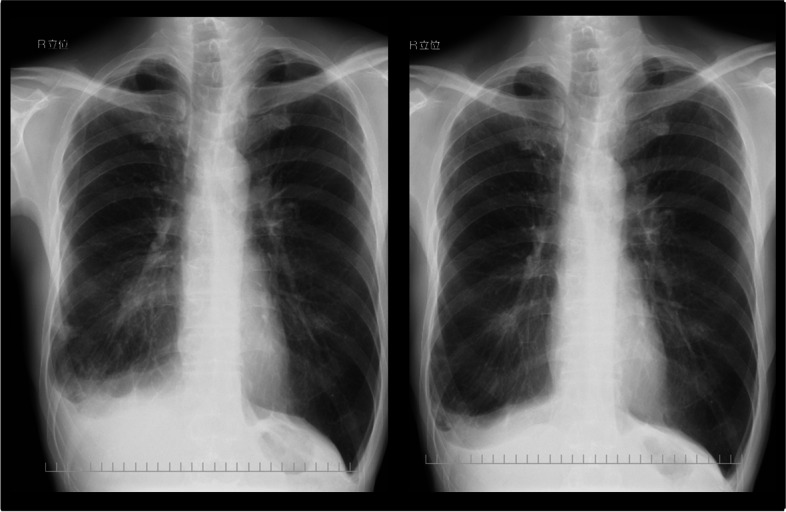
Chest roentgenogram before and after osimertinib treatment. Chest roentgenogram at the initial diagnosis showed a 3 cm mass in the right lower lung field (left). Chest roentgenogram after 4 months’ osimertinib treatment showed an apparent shrinkage of the mass (right)
